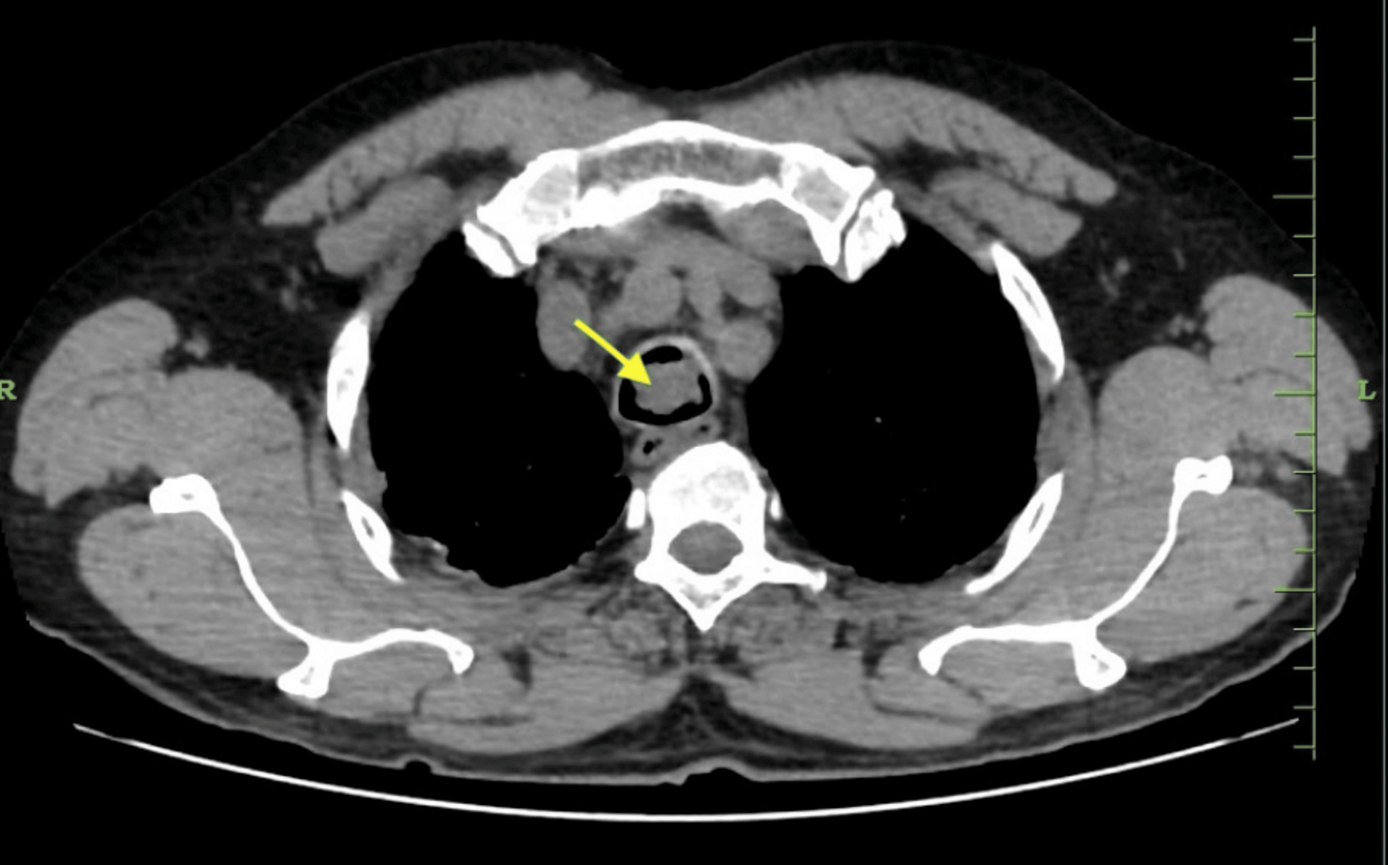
Contrast-enhanced computed tomography (CECT) showing a mass in the trachea (yellow arrow)
